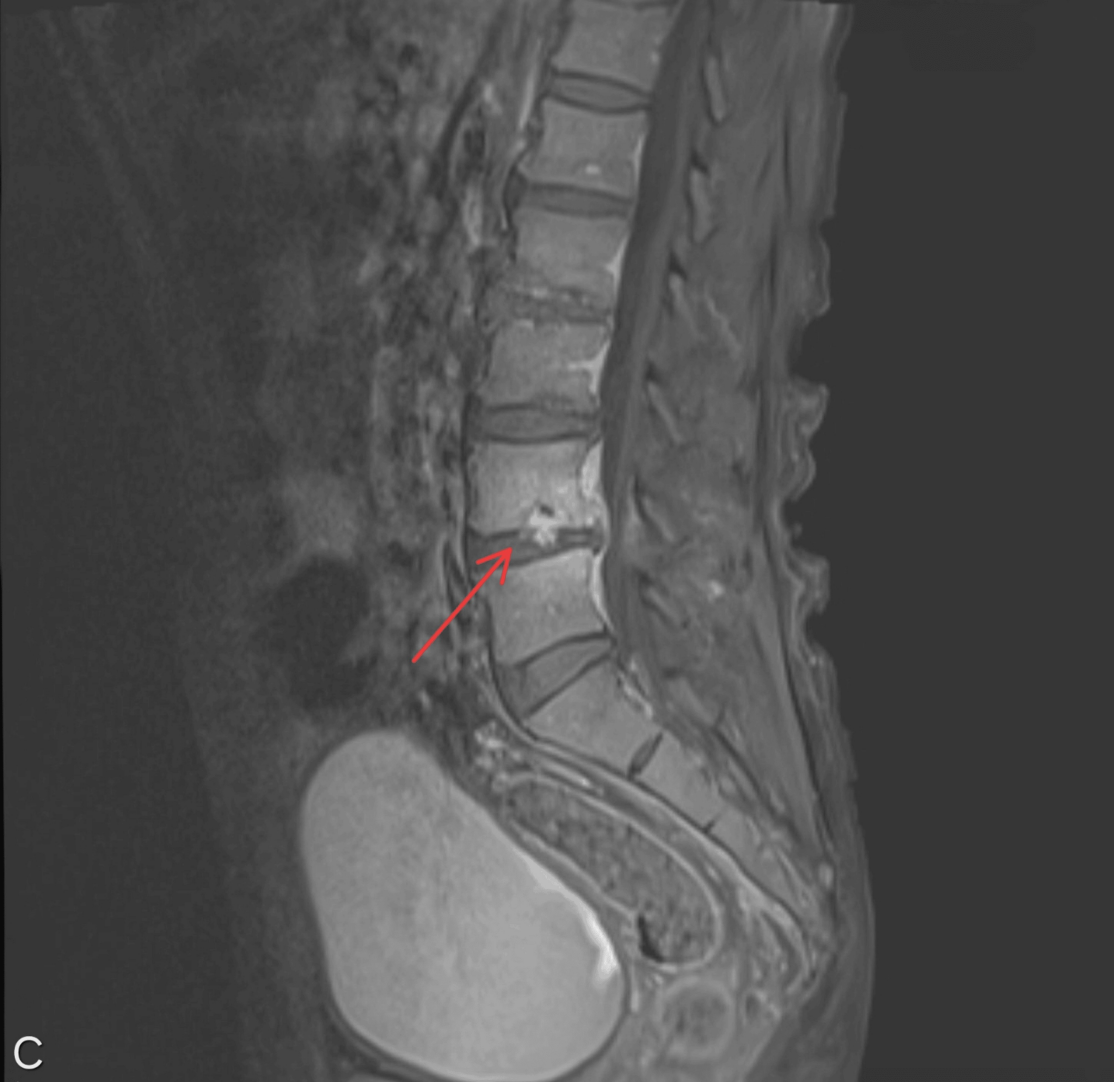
MRI of the lumbar spine with the red arrow showing evolving osteomyelitis at the level L4-L5
